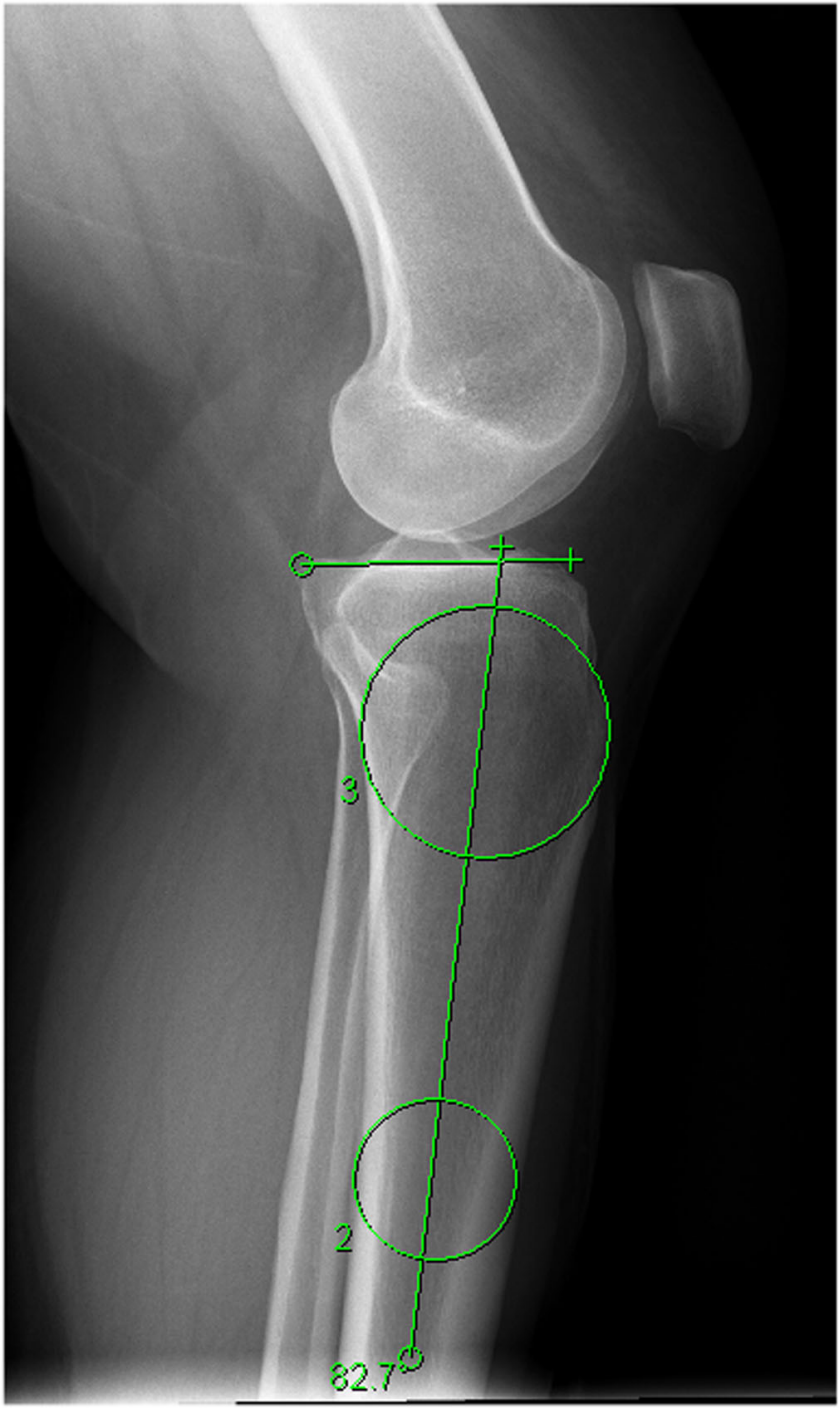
Measurement of PTS using a short knee radiograph. The PTS was calculated by the angle between a line tangential to the medial tibial plateau and the proximal anatomic axis of the tibia that was determined by a line passing through the mid‐cortical diameters of the tibia at a point 5 and 15 cm distal to the joint. PTS, posterior tibial slope.
